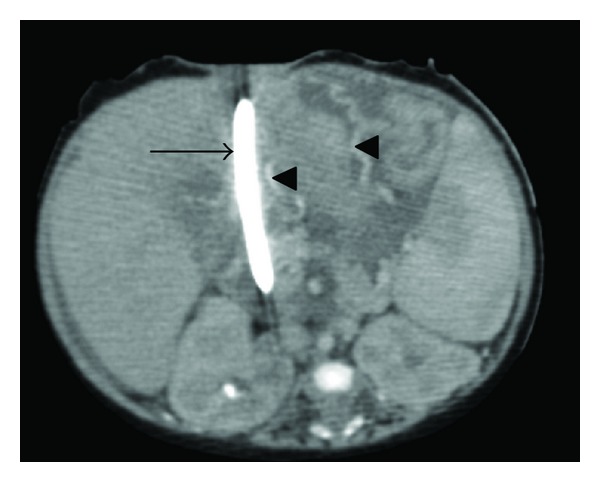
Post IV contrast CT scan in the transaxial plane. Arrow heads point to venous collaterals. Large arrow points to gastrostomy tube.
